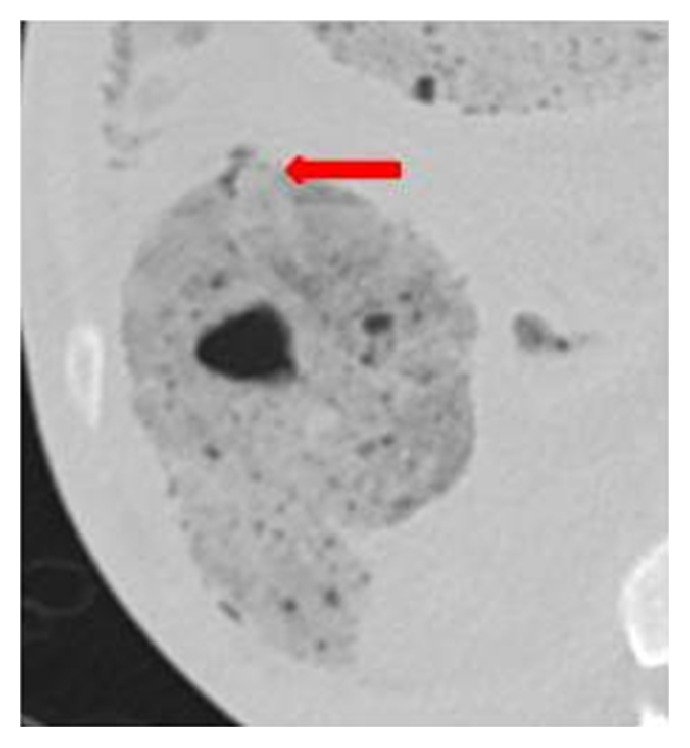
Axial CT image on lung windows (small field of view) confirming the perforation site (arrow).
